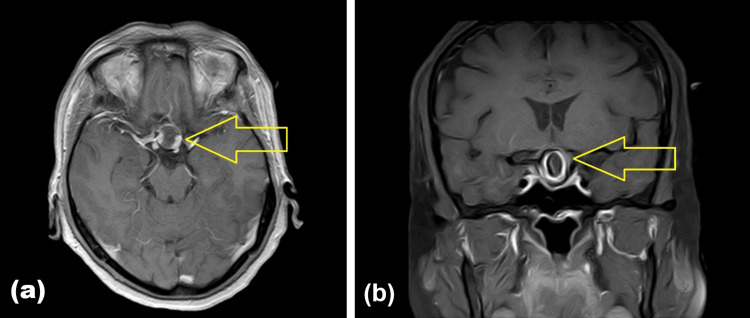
Magnetic resonance imaging (MRI) of the brain. (A) Axial T1-weighted post-contrast image demonstrating a sellar-suprasellar lesion with peripheral rim enhancement and a relatively hypointense central component (arrow). (B) Coronal T1-weighted post-contrast image demonstrating a doughnut-shaped lesion with suprasellar extension, pituitary stalk effacement, and abutment of the optic chiasm (arrow).
